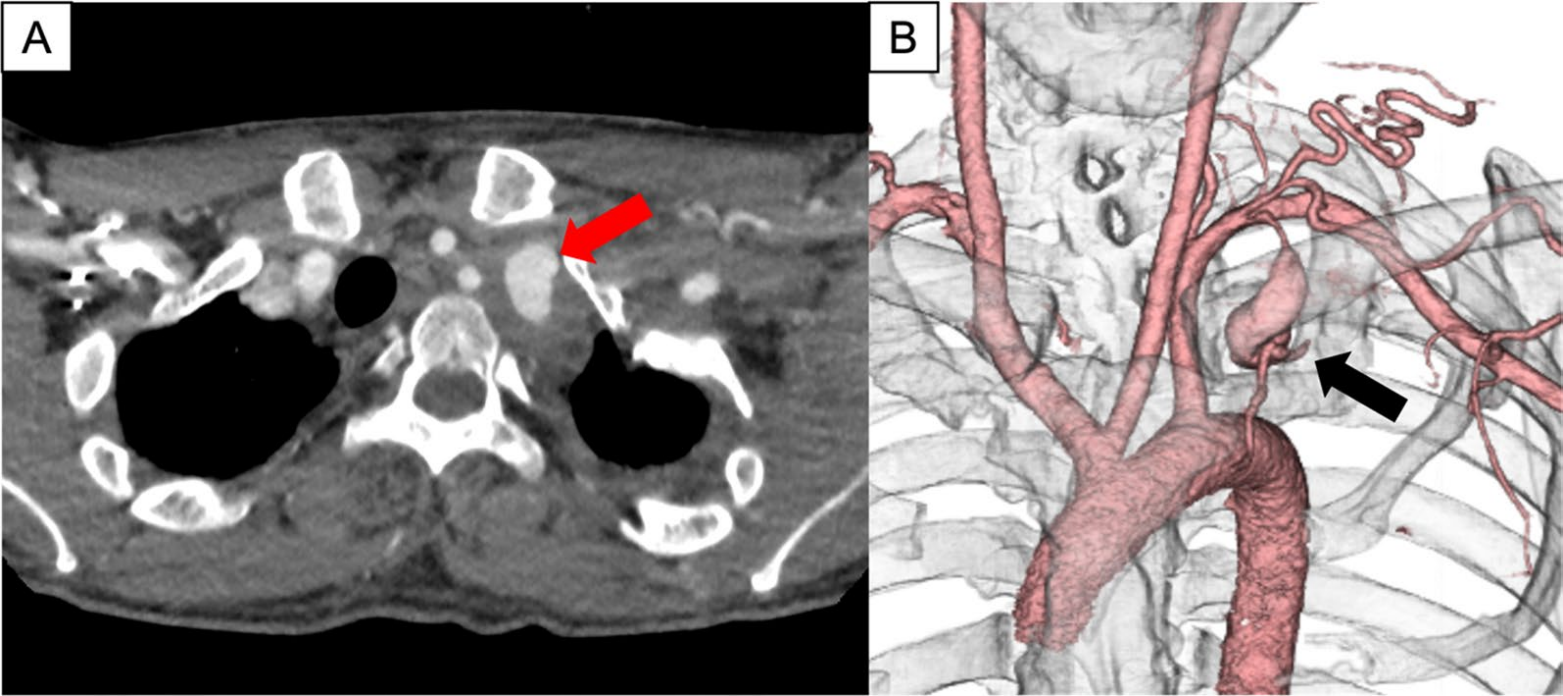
Computed tomography scan. In the axial image from the computed tomography scan, the red arrow indicates the left internal thoracic artery aneurysm (A). In the three-dimensional computed tomography reconstruction, the black arrow indicates the left internal thoracic artery aneurysm (B)
